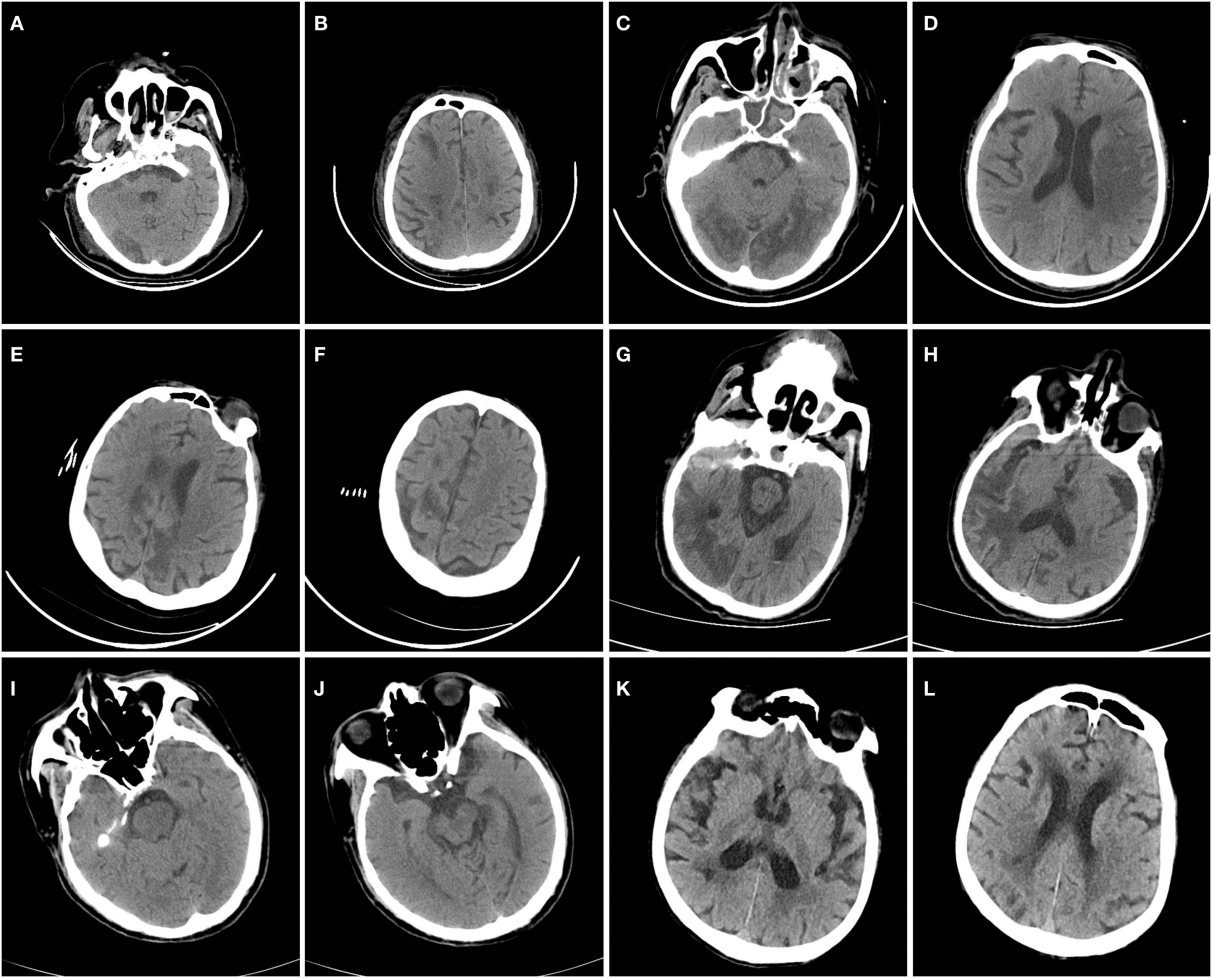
Head CT scans of coronavirus disease 2019 patients with acute ischemic stroke. In Case 1 (A,B), head CT revealed low-density lesions in the right occipital lobe and bilateral frontal and parietal lobes. In Case 2 (C,D), head CT revealed low-density lesions in the bilateral occipital and temporal lobes and the left hemisphere. In Case 3 (E,F), head CT revealed low-density lesions in the bilateral frontal and parietal lobes. In Case 4 (G,H), head CT revealed low-density lesions in the right hemisphere. In Case 5 (I,J), head CT revealed low-density lesions in the left midbrain. In Case 6 (K,L), head CT revealed low-density lesions on the right side of the periventricular area.
